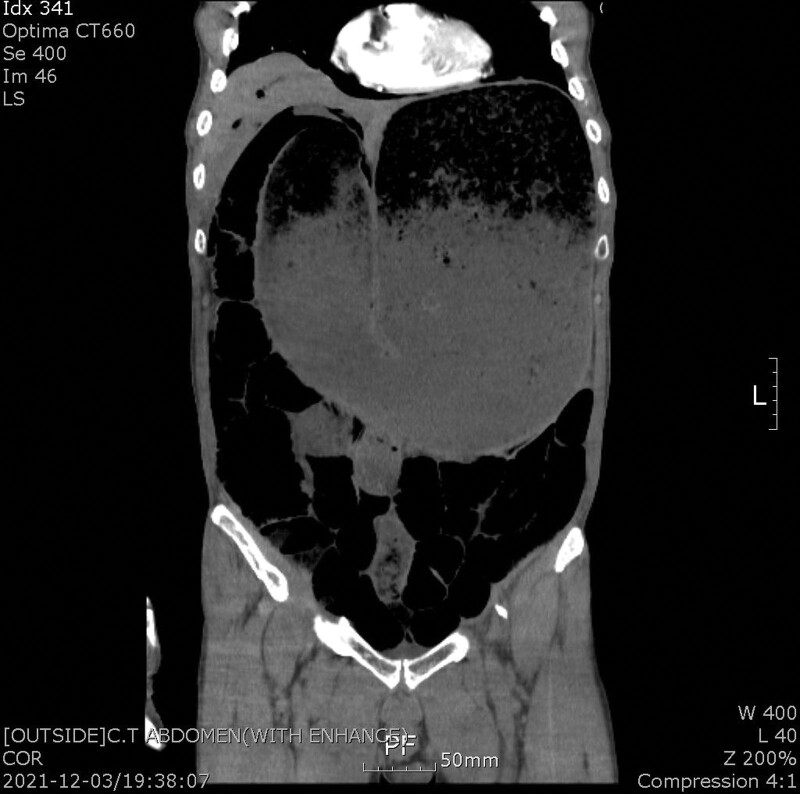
Coronal computed tomography image of the patient showing severe gastric distension resulting in suspicious cardiac deviation and hepatic congestion.
